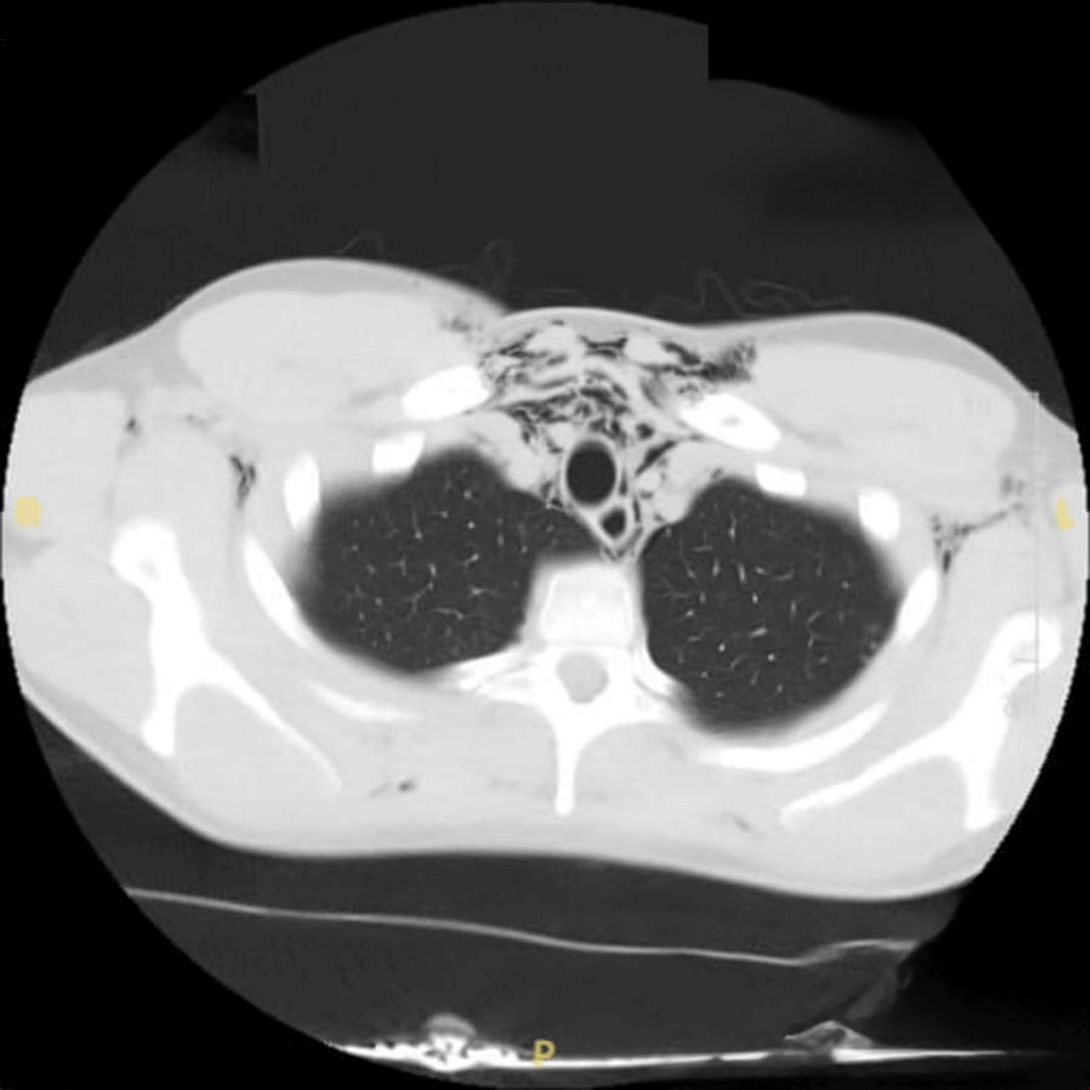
Computed tomography (CT) of the thorax showing moderate pneumomediastinum with surgical emphysema.
